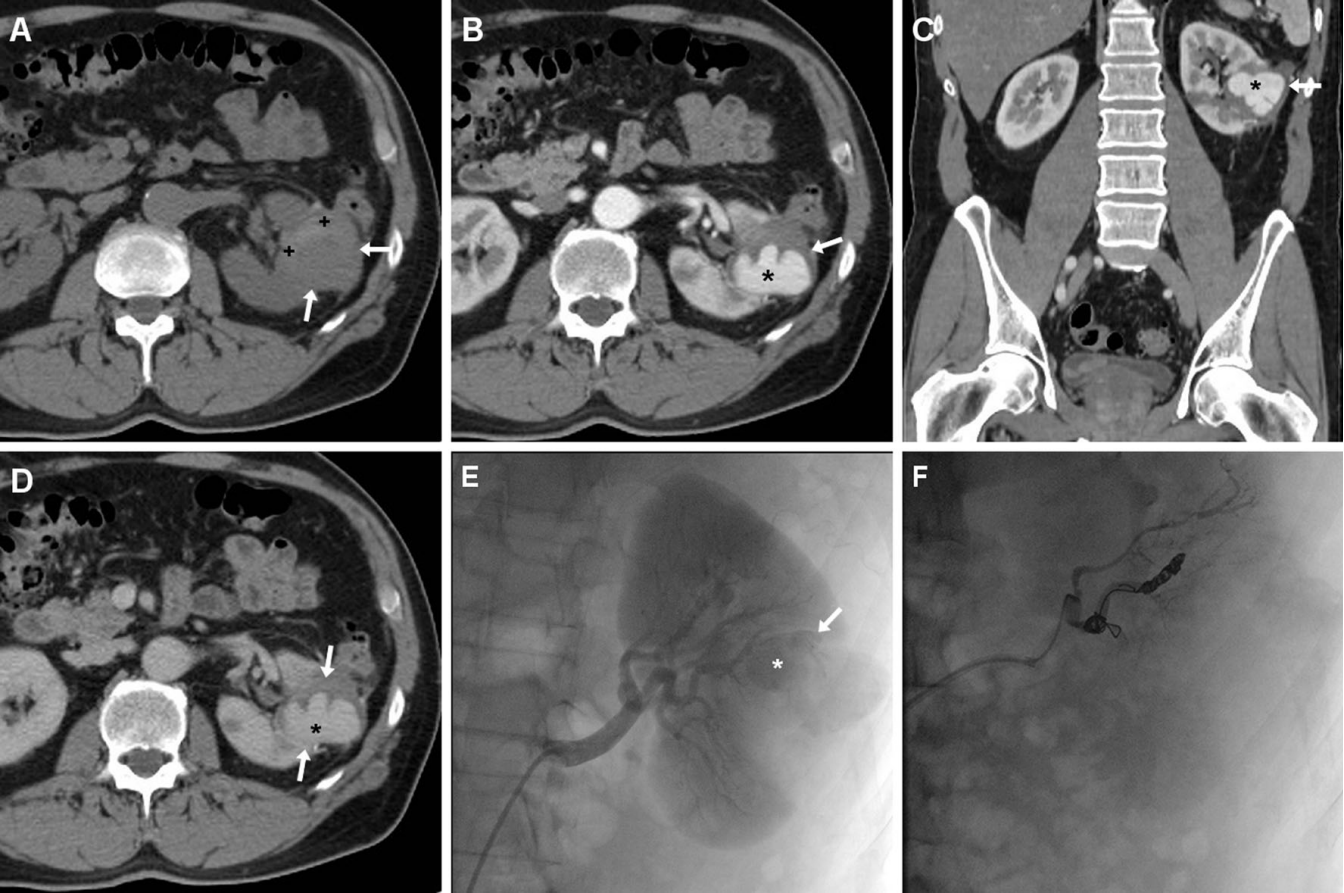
Postoperative gross haematuria in a 55-year-old man 5 weeks after enucleation of a left-sided, 3.7-cm, pT1a clear-cell RCC including the calyceal opening. Unenhanced CT images revealed a 4.5-cm, inhomogeneous, roundish renal mass (*arrowheads*) with non-dependent hyperattenuating material (+), suggesting thrombus. After intravenous contrast, corticomedullary (b, c) and nephrographic (d) CT images showed perfusion (*) synchronous to arterial vessels of most of the same lesion, consistent with pseudoaneurysm. Renal angiography (e) confirmed roundish lesion (*arrow*) occupied by haemorrhagic focus (*) from the distal portion of the anteromedial branch, corresponding to the site of recent tumour excision. Super selective catheterisation and embolisation with coils (f) allowed the bleeding to be stopped
